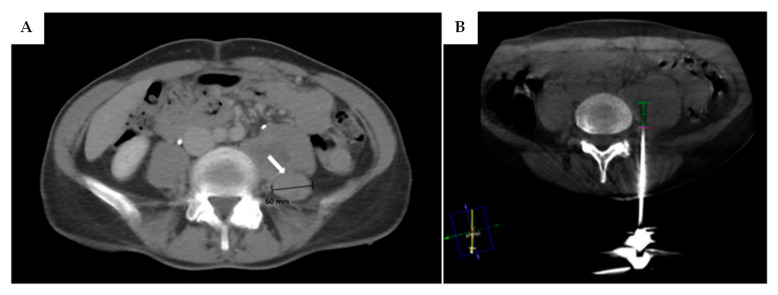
(A) CT scan of the low abdomen of patient 3 showing the round neoplasm (white arrow) located behind the left psoas muscle at L4-L5 level, measuring ~60 mm in diameter. (B) CT-guided biopsy was performed to rule out the hypothesis of a malignant lesion, which it was later confirmed to be.
